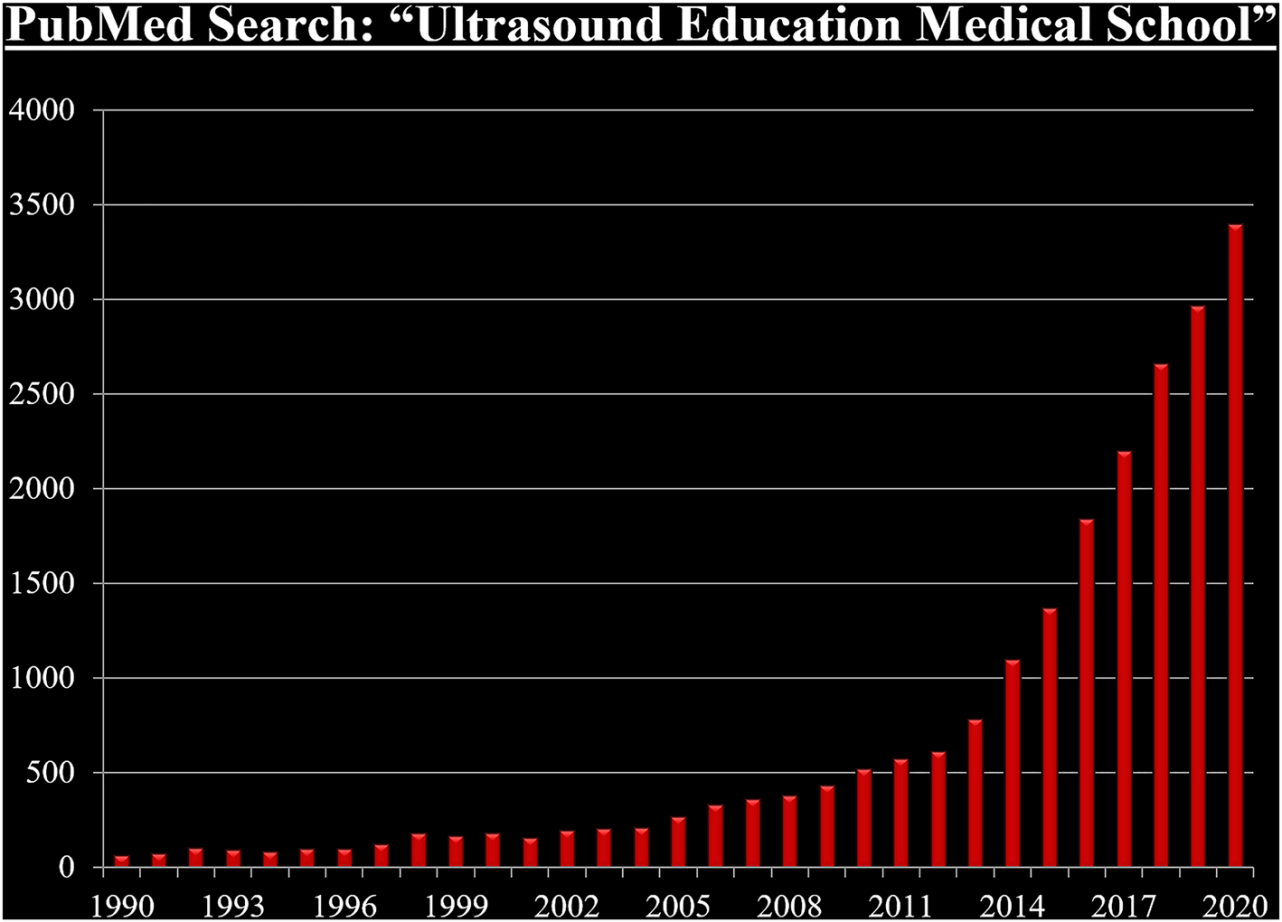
A PubMed search of articles using *ultrasound education medical school* as the query
